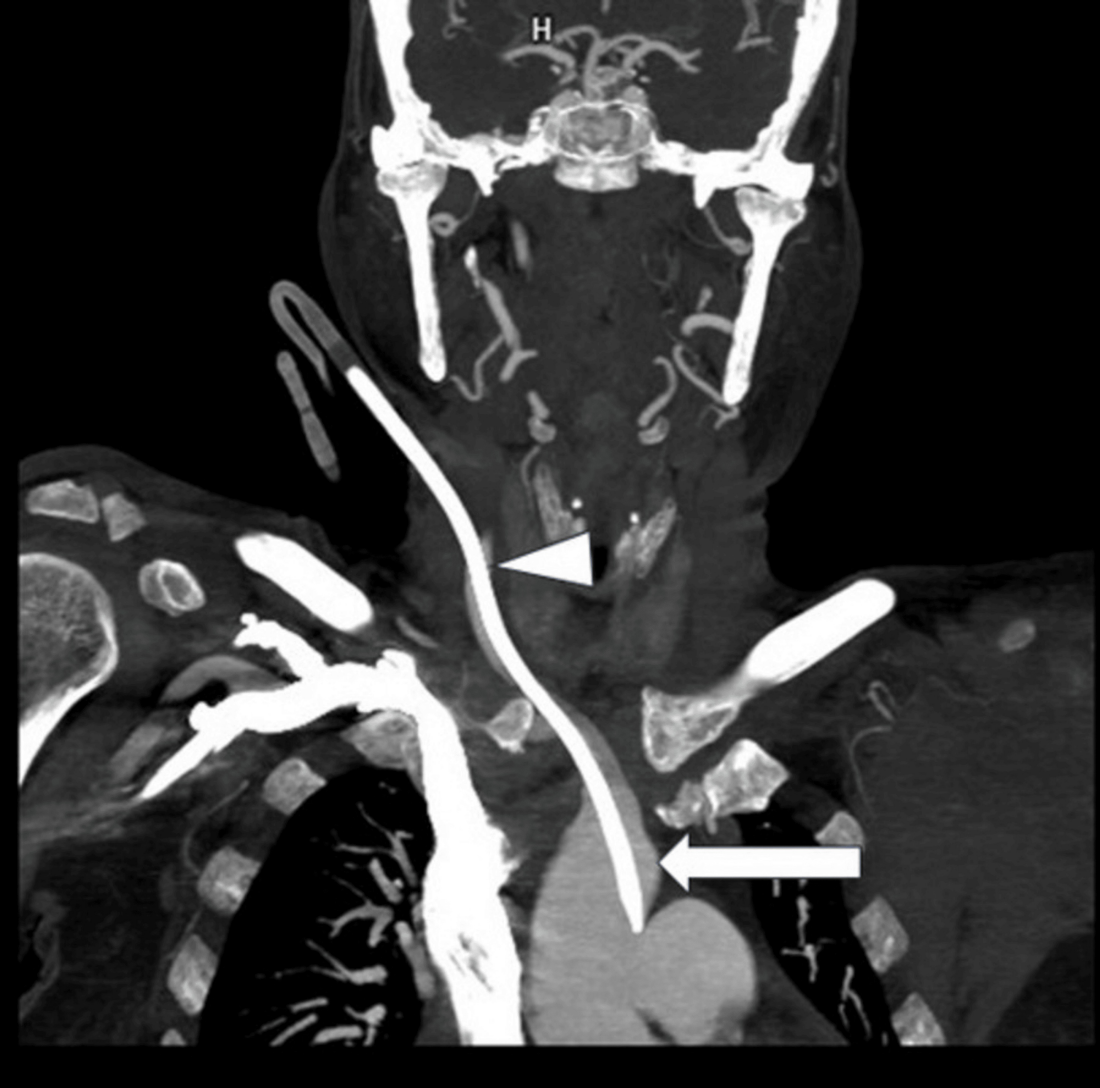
Coronal MIP-reconstructed CT angiography image showing the dialysis catheter in the right common carotid artery (arrowhead), extending up to the aortic arch (arrow). MIP: maximum intensity projection
